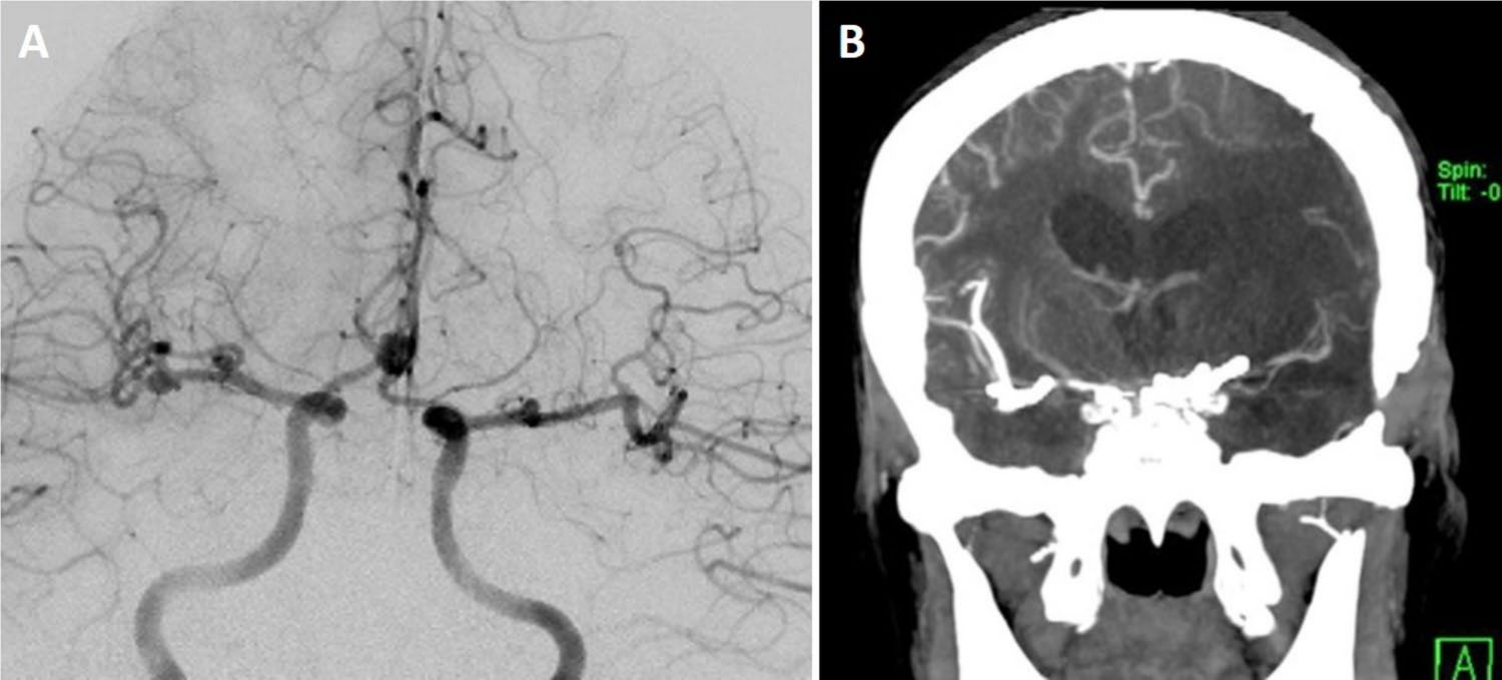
A DSA shows four aneurysms, B CT angiogram shows infarction in the MCA territory despite normal blood flow in the left MCA
